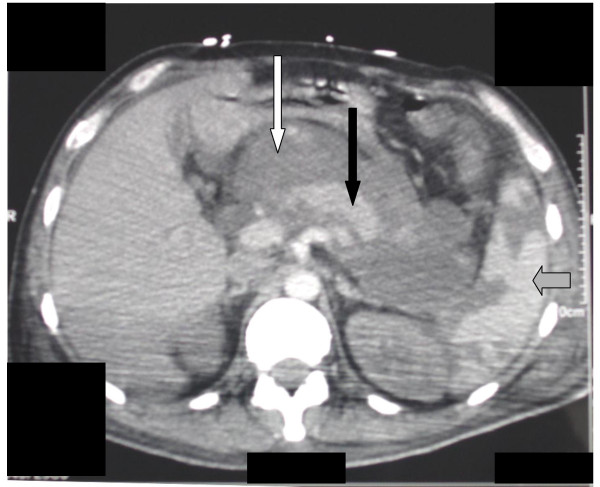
Abdominal CT scan showing the presence of large and heterogeneous hypodensities (open arrow), surrounding the pancreas (filled arrow) and mildly enhanced following contrast infusion. The spleen (gray arrow) was patchy, demonstrating ischemic zones.
